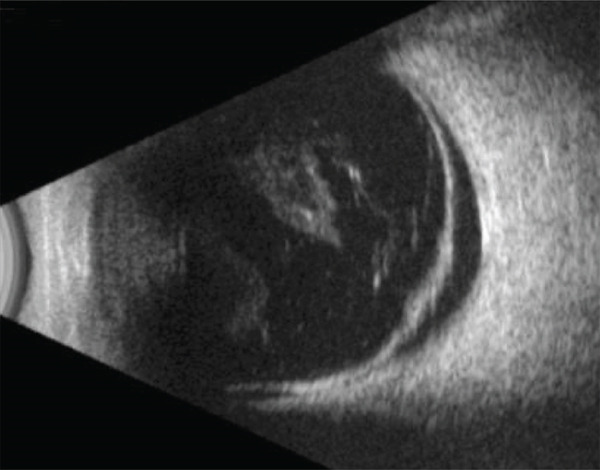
Preoperative superonasal longitudinal B‐scan ultrasonography of the right eye demonstrating retinal detachment extending to the macular region. Vitreous strands with vitreoretinal adhesions are observed in the midperipheral retina.
